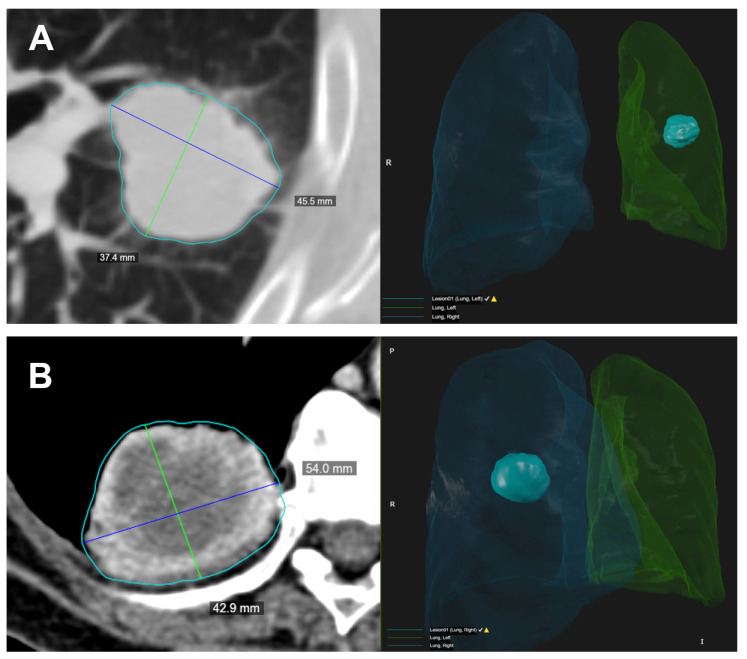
Two examples (A,B) of the semi-automated identification of the target lesion. On the left, the CT image with the lesion segmentation (light blue contour) and the longest diameters measured on the lesion volume. The blue lines represent the longest long axes and the green lines represent the longest short axes on the axial direction. On the right, it is possible to observe the 3D rendering of the lesion volume and its location inside the automatic lung segmentation.
